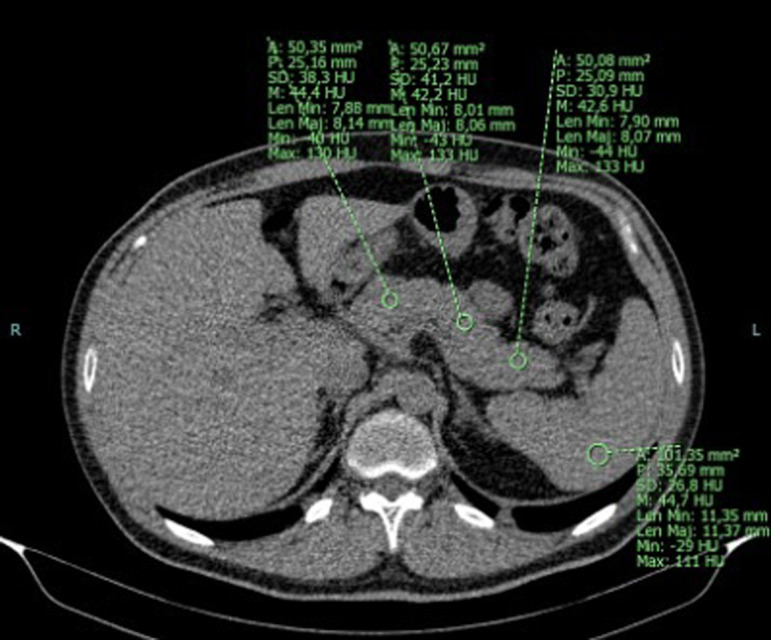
An example of the measurement of attenuation values of liver and spleen on non-enhanced computed tomography by using region of interest.
